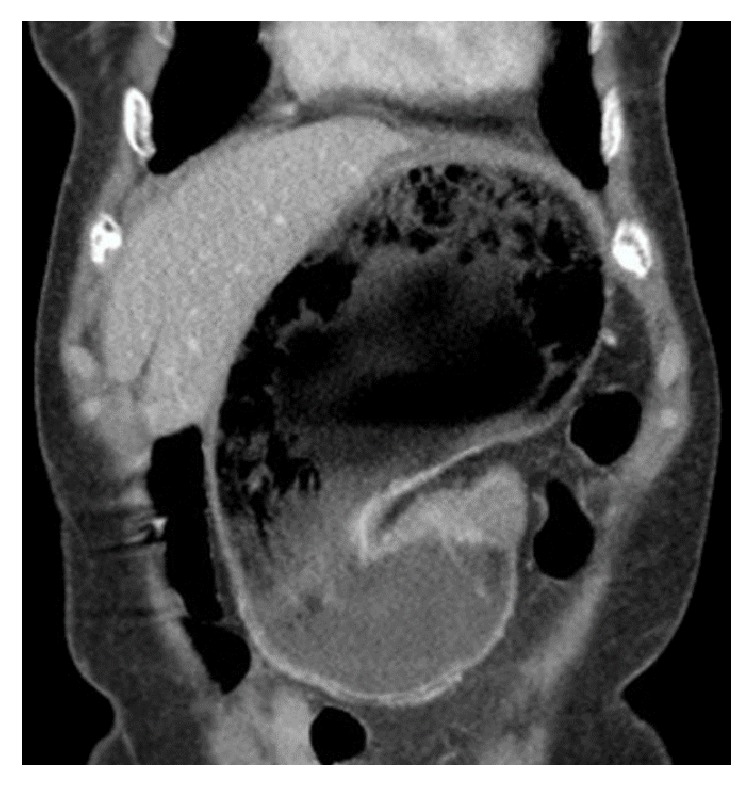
CT of abdomen and pelvis with contrast showing a markedly distended abdomen without focal wall thickening. The J-shaped stomach is rotated along its long axis, displaying a gastric volvulus. Fluid, consistent with blood, is seen tracking along the paracolic gutters and the pelvis.
